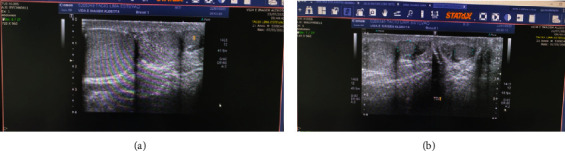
Ultrasound of the right hemiscrotum (a, b).
